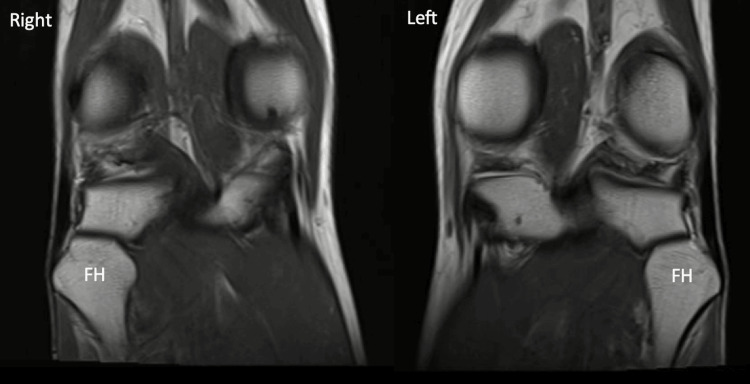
T2-weighted magnetic resonance imaging (MRI) scan of the patient's right and left knee. The prominent fibular heads (FH) are labelled and measure 30 mm in diameter.
